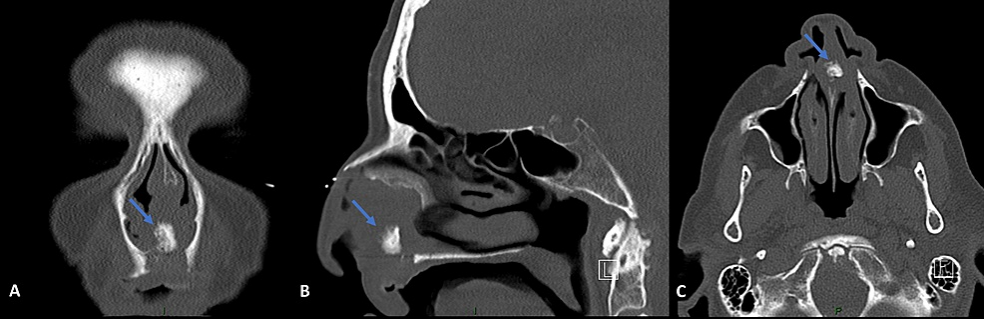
Preoperative paranasal sinuses CT scan. Paranasal sinuses CT scan showing an intraseptal submucosal mass with a central calcification (arrow) in coronal (A), sagittal (B), and axial (C) views.
